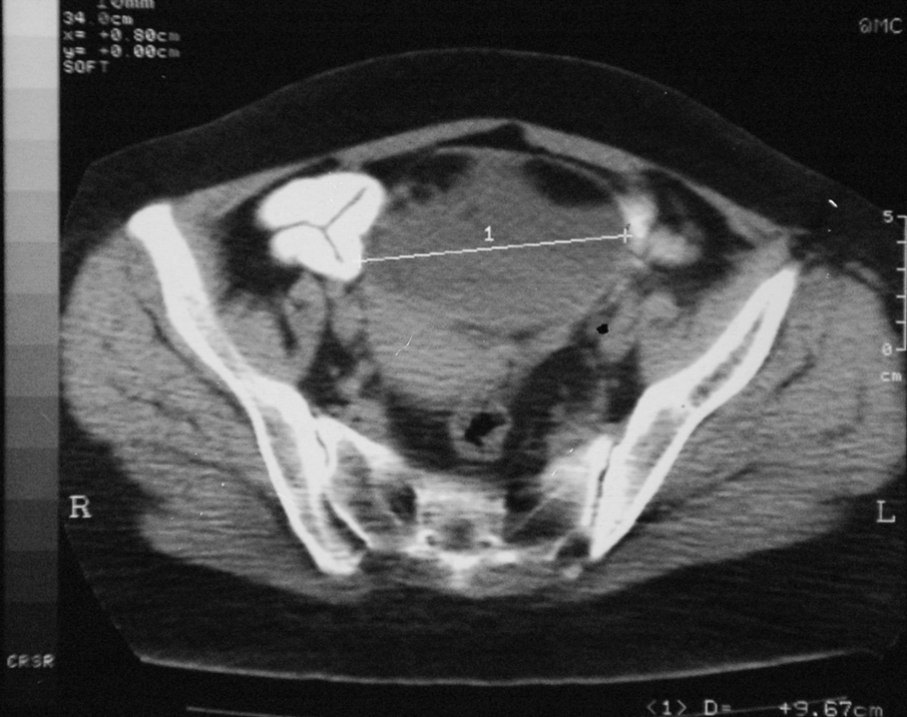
Abdominal computed tomography scan: a heavily triple tissular mass with greasy and osseous constituent (= 10 cm).
